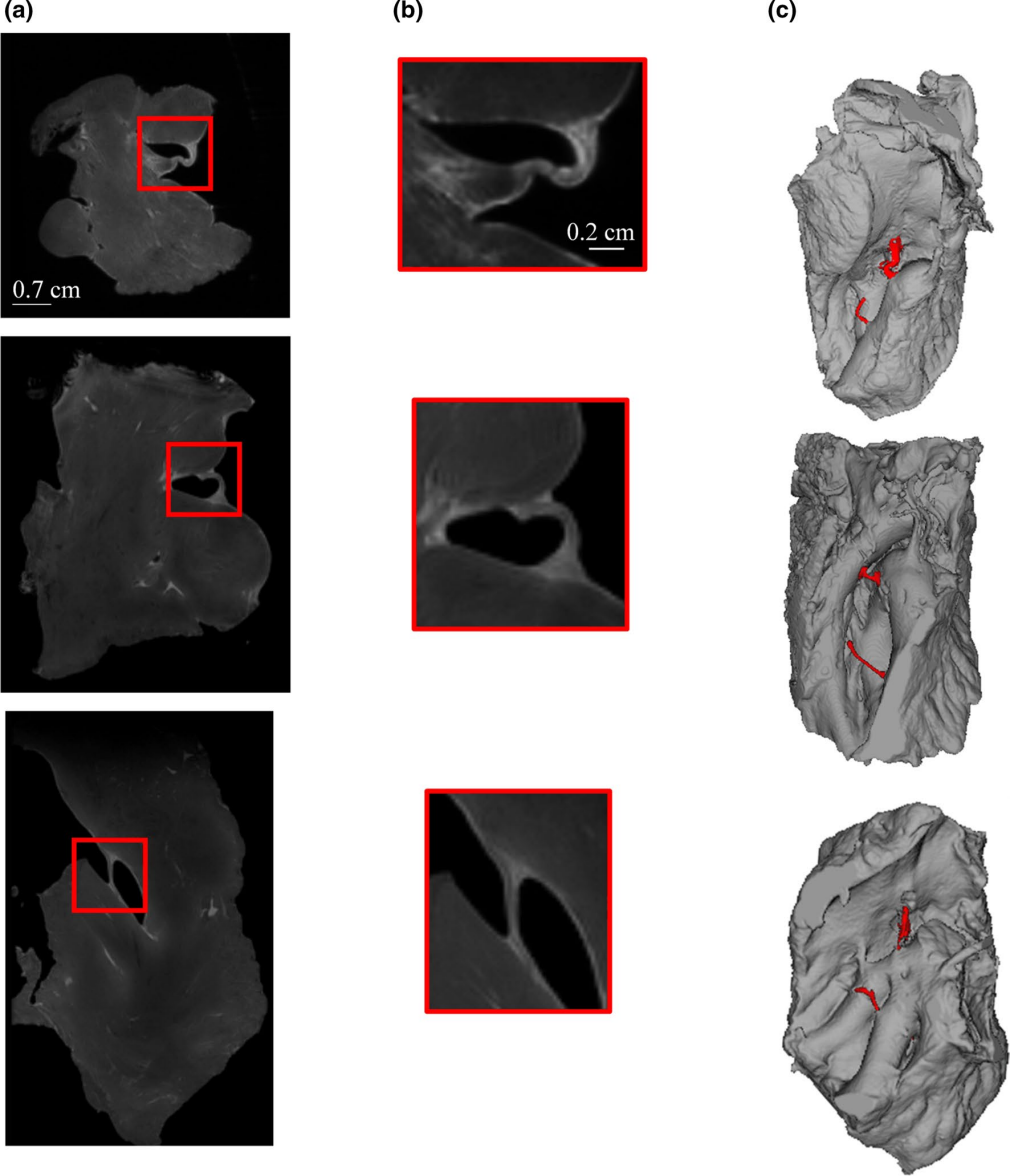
a Transverse slices orientated from the 3D volume at an isotropic resolution of 150 μm using MT MRI for sample 1, 2 and 3. Insertion points of the fiber into the myocardium are expanded in the red box and zoomed (b). c Fibers are segmented in red on a 3D volume rendering using ItkSnap
